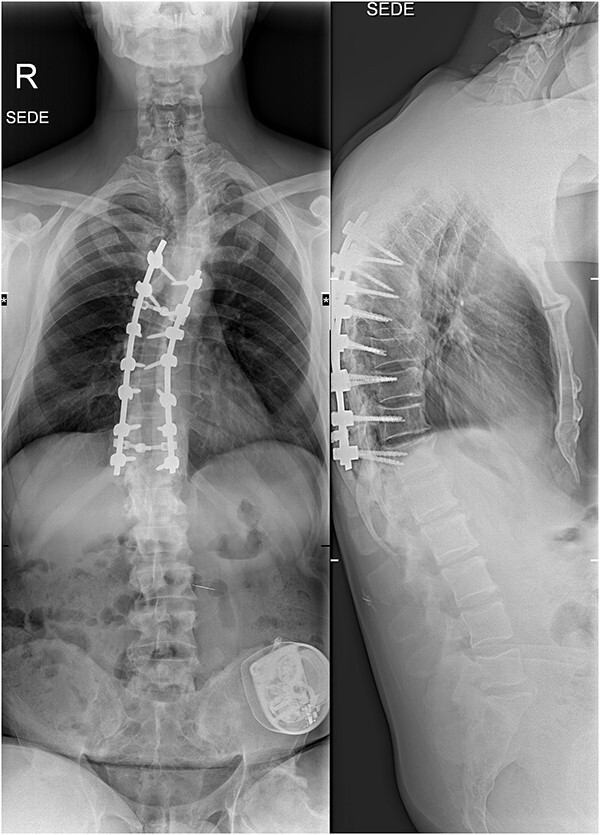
X-rays at the final follow-up 4 years after the surgery. Anteroposterior view (left) and lateral view (right).
